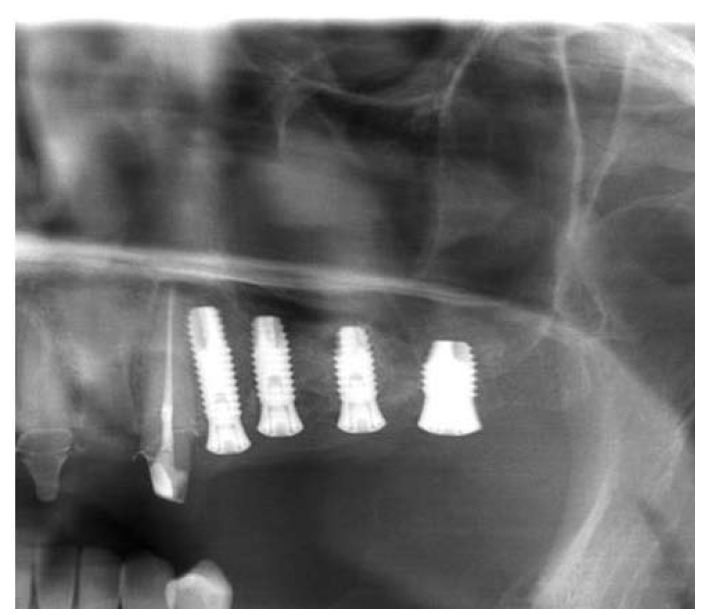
Postoperative panoramic X-ray view.
